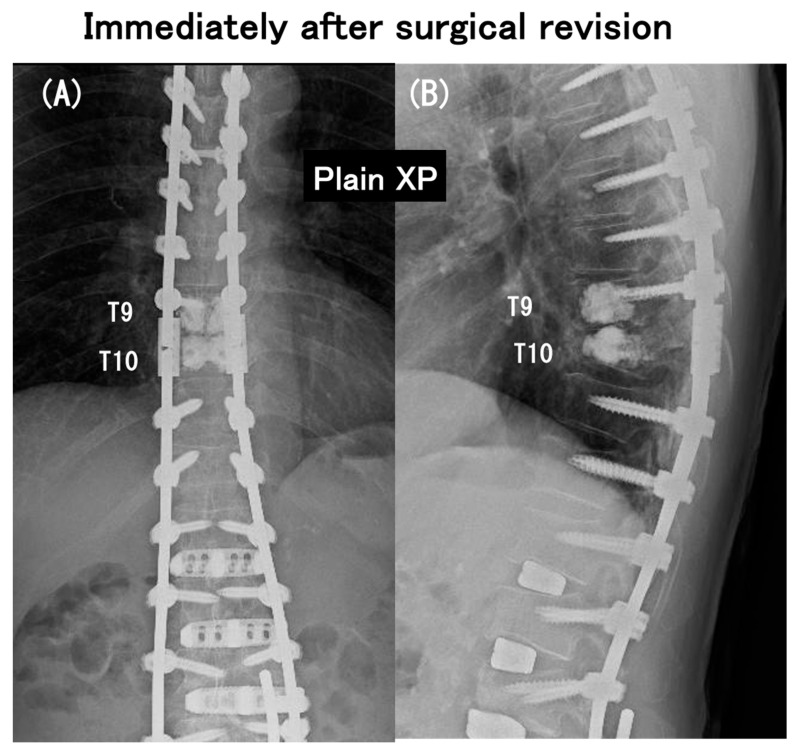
Coronal (A) and sagittal (B) radiographs obtained at an early postoperative period of urgent surgical revision extending the posterior instrumented fusion proximally to T5 with spinal cord decompression.
